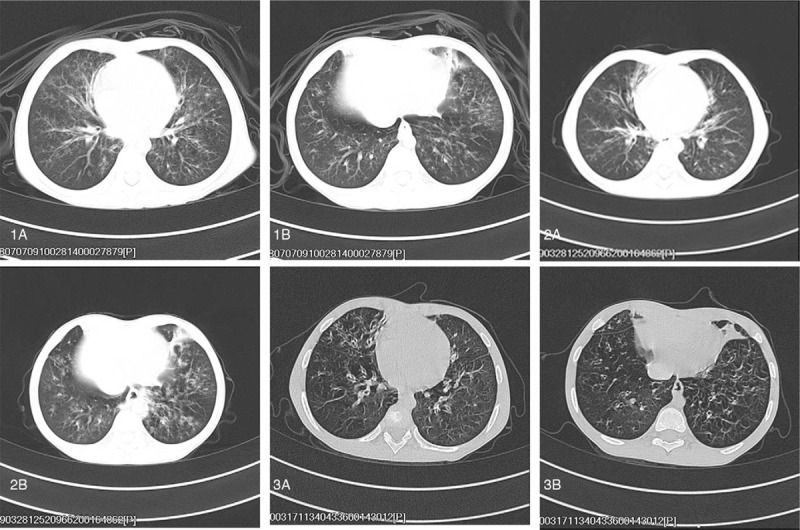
Chest high resolution computed tomography (HRCT): July 1, 2018: bilateral lung pneumonia with partial mild bronchiectasis. April 2, 2019: bronchiectasis was seen in the bilateral lungs. March 3, 2020: emphysema associated with bronchiectasis in the bilateral lungs.
